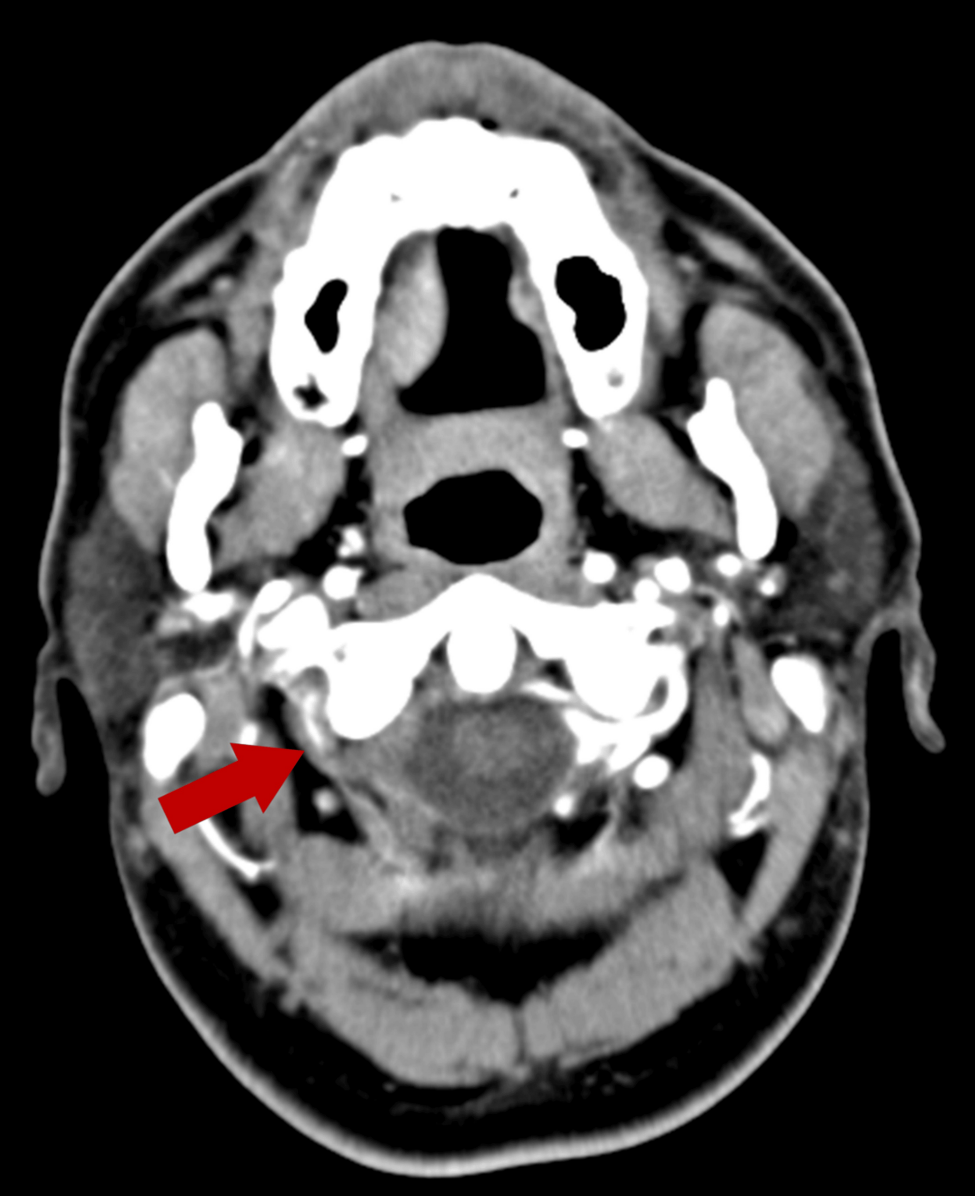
CT angiography scan CT angiography demonstrates tapering and focal occlusion of the distal cervical segment (V3) of the right vertebral artery (red arrow), consistent with arterial dissection. The absence of contrast opacification beyond this point supports a diagnosis of flow-limiting intramural hematoma. This finding correlates with the patient’s posterior circulation ischemic symptoms and localizes the vascular injury site.
